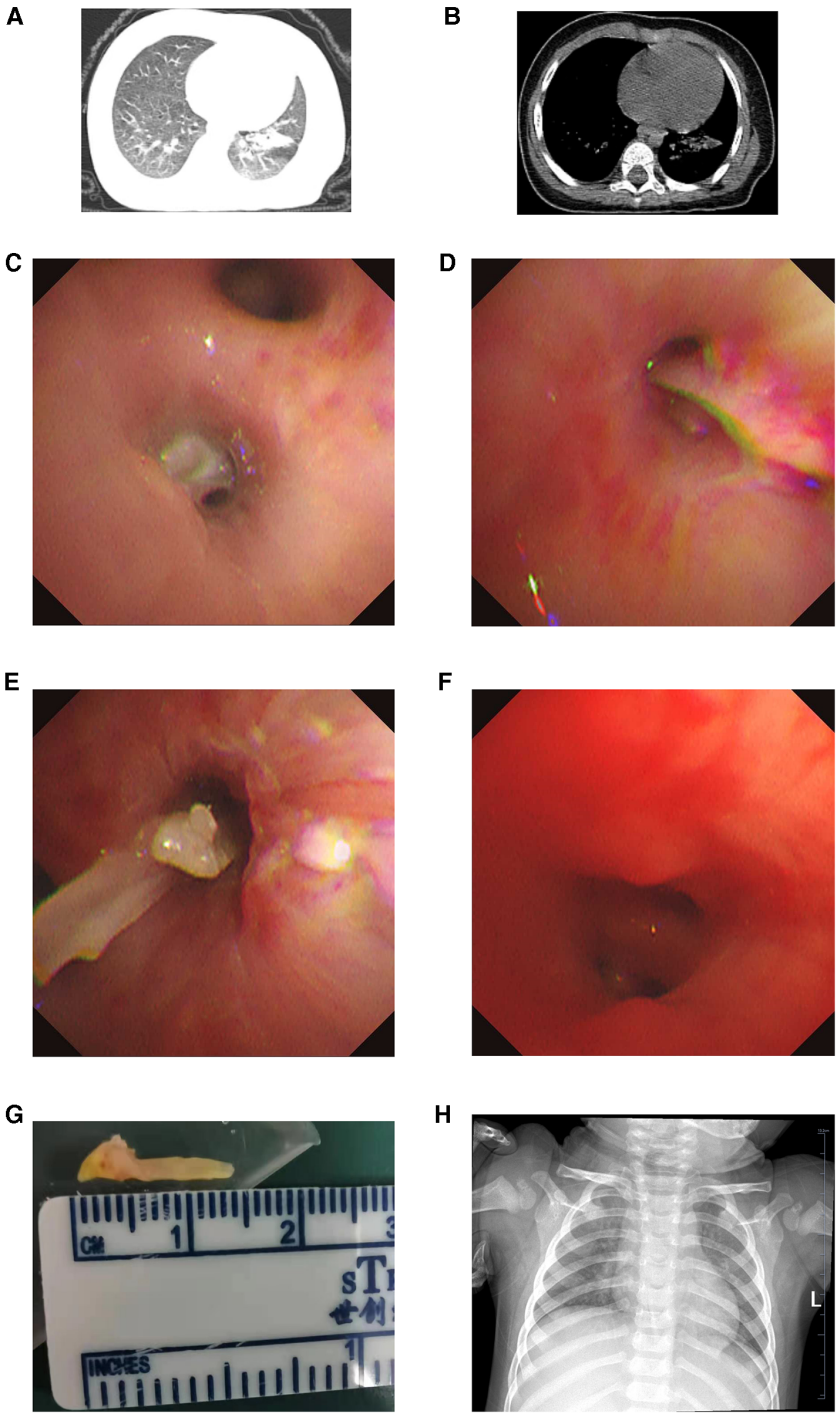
Lung (A) and mediastinum (B) window of chest CT showing atelectasis in the left lower lobe; (C) under flexible bronchoscopy, residual FB lodged in the outer basal segment of the left lower lobe bronchus prior to extraction; (D,E) residual fish bone pulled by grasping forceps through flexible bronchoscope; (F) the aspect of the outer basal segment of the left lower lobe bronchus after FB removal; (G) the residual fish bone measuring 1.5 cm in length; (H) the aspect of chest x-ray on discharging. CT, computed tomography; FB, foreign body.
